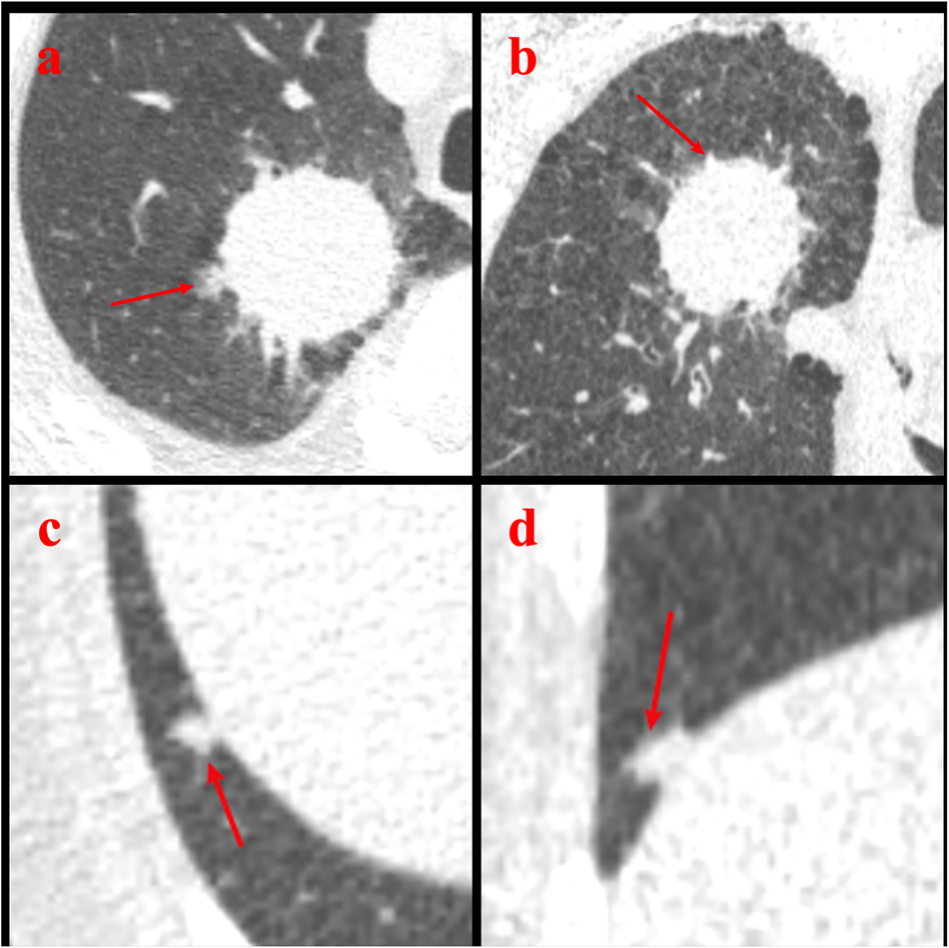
Examples where AI did not detect the risk-dominant nodules determined by reference standard. Example 1. LDCT LCS images from a 65-year-old male. a Axial (D45f/1 mm, lung window) and (b) coronal (D45f/1 mm, lung window) LDCT images show a vessel-attached lesion in the right upper lobe. The reference standard determined this lesion as the risk-dominant nodule and classified it as noncalcified solid (total volume = 39,810.0 mm^3^). However, AI on all four reconstruction settings did not mark this lesion as a nodule, because the lesion larger than 30,000 mm^3^ in volume was regarded as a mass and not segmented by the AI algorithm. Example 2. LDCT LCS images from a 67-year-old male. c Axial (D45f/1 mm, lung window) and (d) coronal (D45f/1 mm, lung window) LDCT images show a pleura-attached lesion in the right lower lobe. The reference standard determined this lesion as the risk-dominant nodule and classified it as noncalcified solid (total volume = 90.0 mm^3^). However, AI on all four reconstruction settings did not mark this lesion as a nodule. LCS, lung cancer screening; LDCT, low-dose CT
